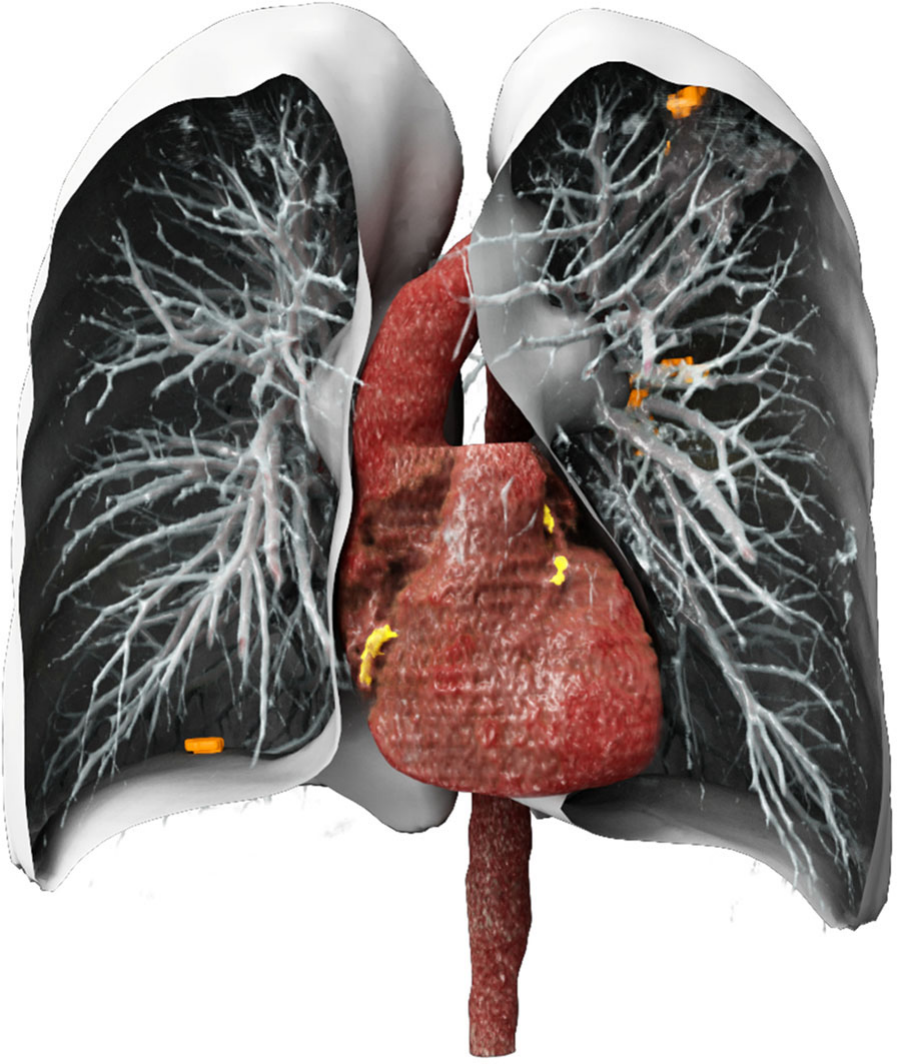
Cinematic volume rendering of the detected coronary calcium (yellow) and lung nodules (orange) along with the lungs, pulmonary vessel, aorta, and heart segmentations from a representative low-dose chest computed tomography
